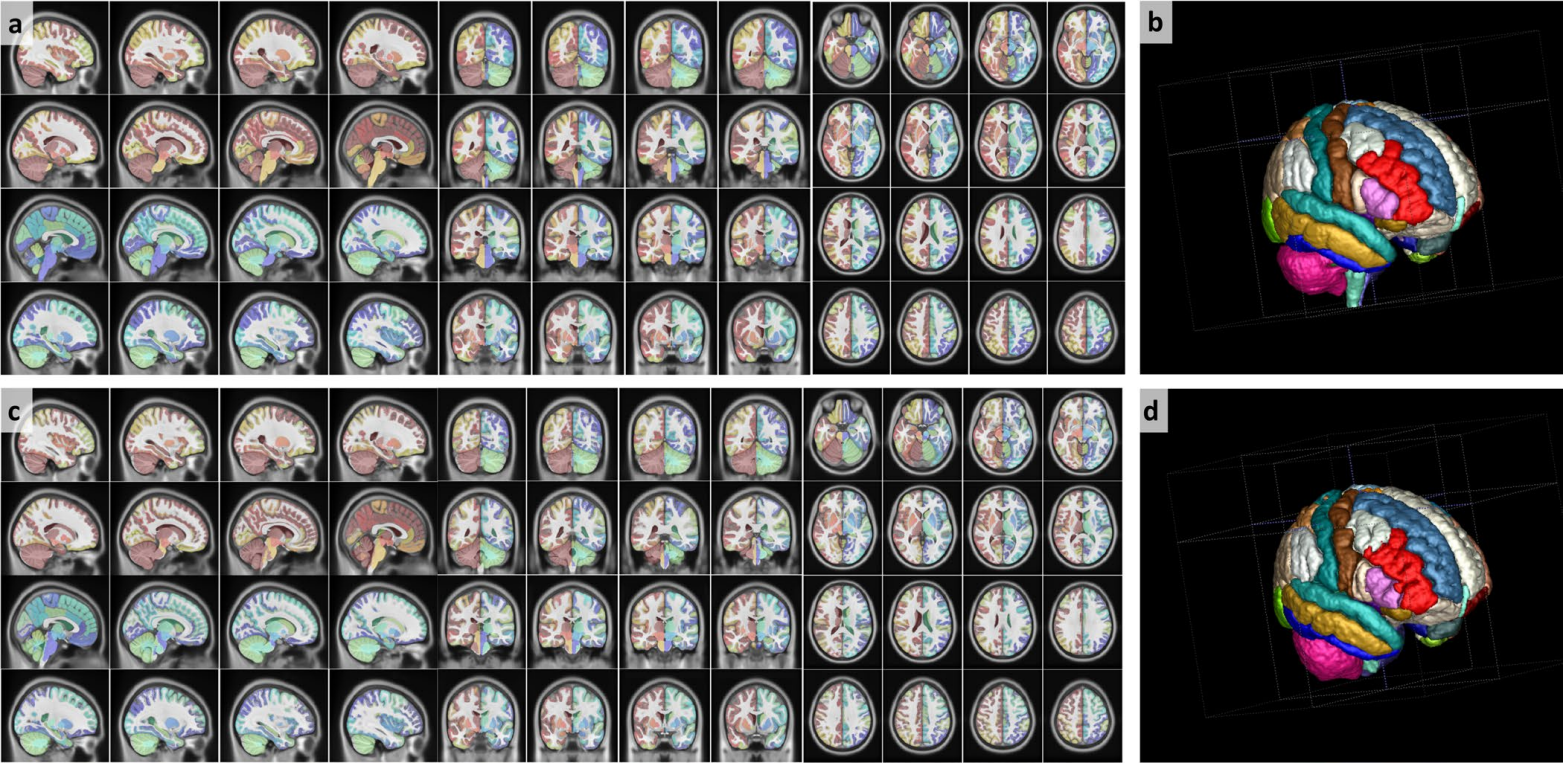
Warped CerebrA atlas (a,b) and Mindboggle-101 atlas (c,d) overlaid on the ICBM152 non-linear 2009 symmetric average MRI template. Note the improved label alignment on the cortical structures.
